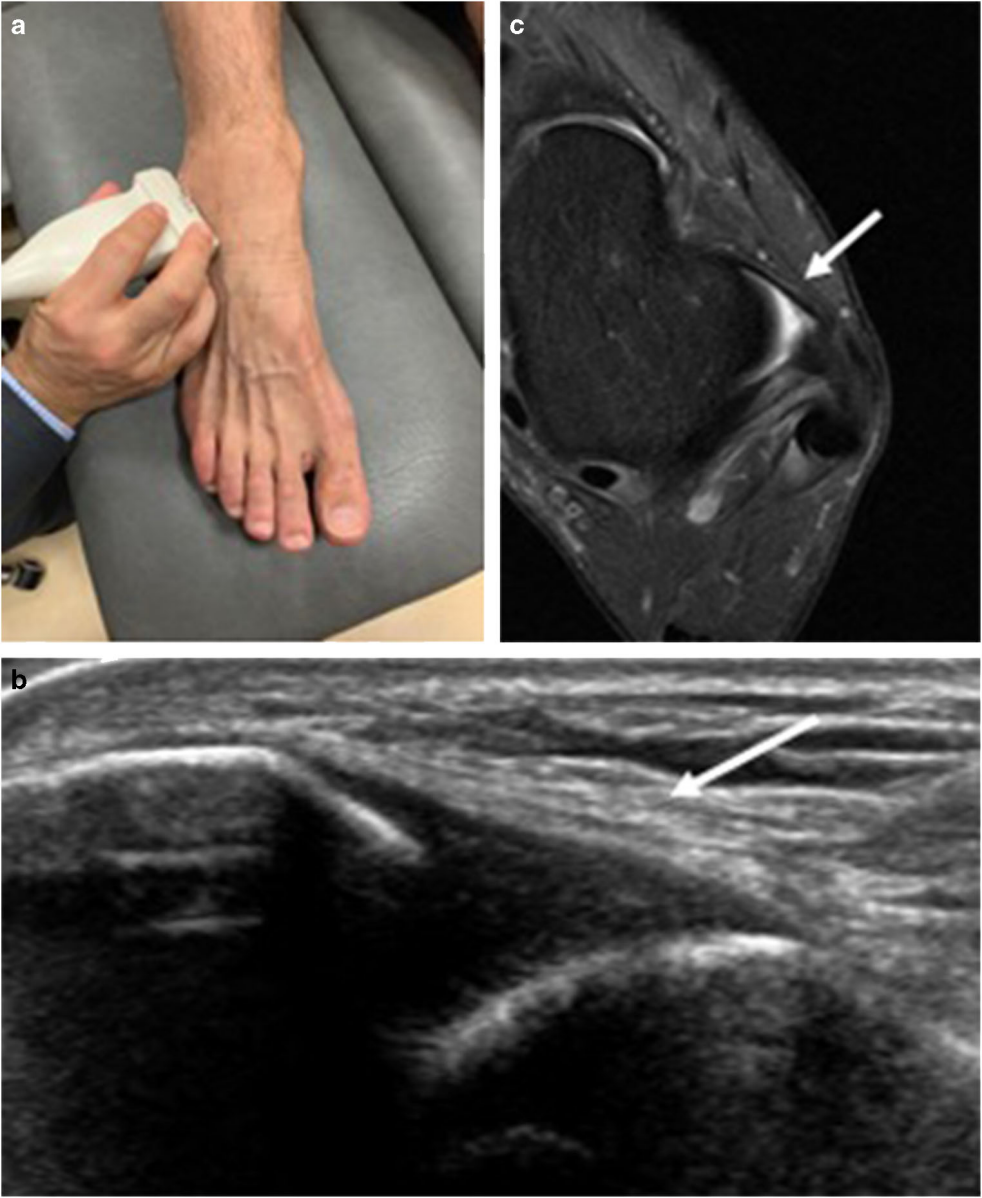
Probe position and (US/MR) imaging results for the anterior talofibular ligament (ATFL). Ultrasound for the diagnosis of anterior talofibular ligament (ATFL) injury. a The ultrasound probe is placed in the transverse plane (longitudinal to the ATFL) anterior to the tip of the lateral malleolus. b Ultrasound findings consistent with an intact anterior talofibular ligament (arrow). c Axial PD-FS image showing an intact anterior tibiofibular ligament (arrow)
